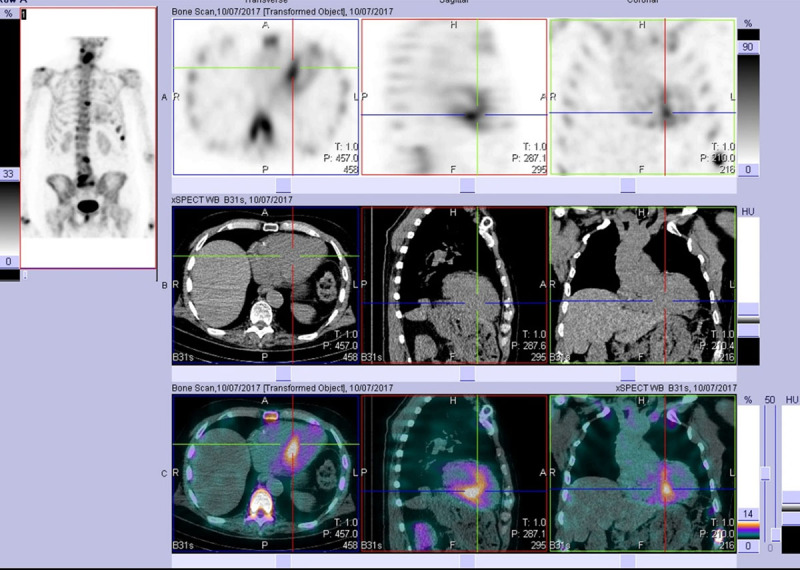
After viewing the planar study, a SPECT-CT was performed. This representative slice in axial, sagittal, and coronal plains (top row = SPECT, middle row = CT, bottom row = merged) confirms the uptake as cardiac and not blood pool. Echocardiogram at the time was normal. The patient developed echo and clinical features of cardiac ATTR amyloid approximately two years later. (Images courtesy of Cabrini Health, Victoria, Australia).
